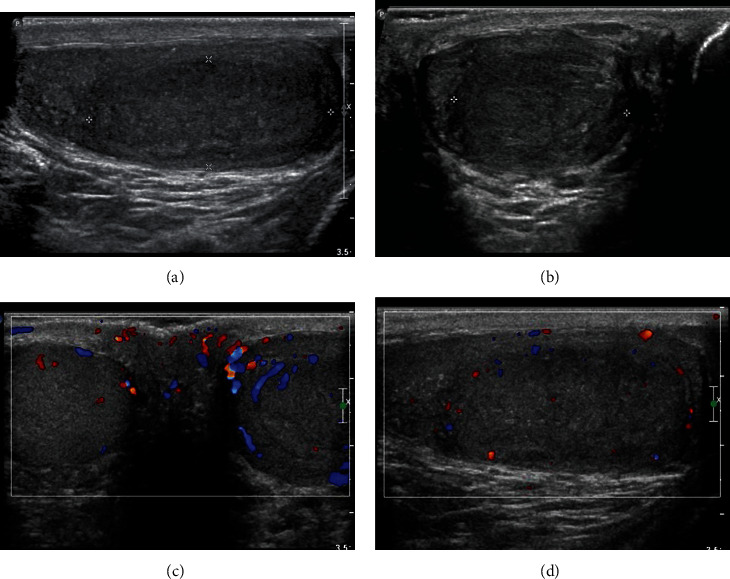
(a, b) US demonstrate a 2.4 × 1.6 × 3.7 cm left intratesticular heterogenous hypoechoic mass ((a) sagittal view; (b) transverse view). (c) Color Doppler transverse view demonstrates asymmetrical enlargement of the left testicle (right side) containing a heterogeneous mass, compare to the right testicle (right side). (d) Mild internal vascularity of the mass in the left testicle.
